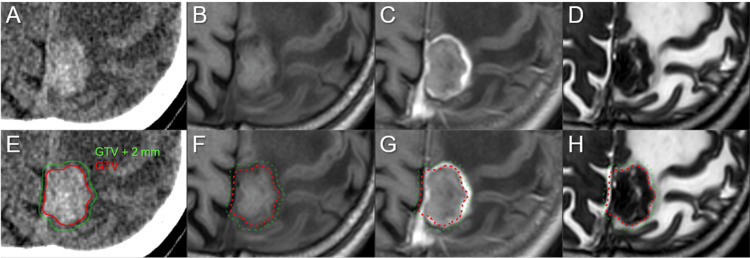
Target definition based on the multi-image co-registration, fusion, and comparison for stereotactic radiosurgery The axial images show non-CE-CT images (A, E); non-CE-T1-WIs (B, F); CE-T1-WIs (C, G); T2-WIs (D, H); and target contours superimposed onto the images (E-H). (A-H) These images are shown at the same magnification and coordinates under co-registration and fusions. The gross tumor volume (GTV) is defined based on a comparison of the visible masses on CT images, CE-T1-WIs, and T2-WIs (CT/T1/T2 matching). The GTV (7.14 cm^3^) is contoured mainly based on the boundaries of the high-density mass on CT images (A, E) and the low-intensity mass on T2-WIs (D, H), excluding the excessive exudation of contrast media (C, G). The GTV + 2 mm structure was generated by adding an isotropic 2-mm margin to the GTV for the evaluation of the dose gradient outside the GTV boundary. (C, G) The boundary of the GTV + 2 mm structure (12.33 cm^3^) almost corresponds to that of the enhancing lesion (11.66 cm^3^). CE: contrast-enhanced; CT: computed tomography; WIs: weighted images
